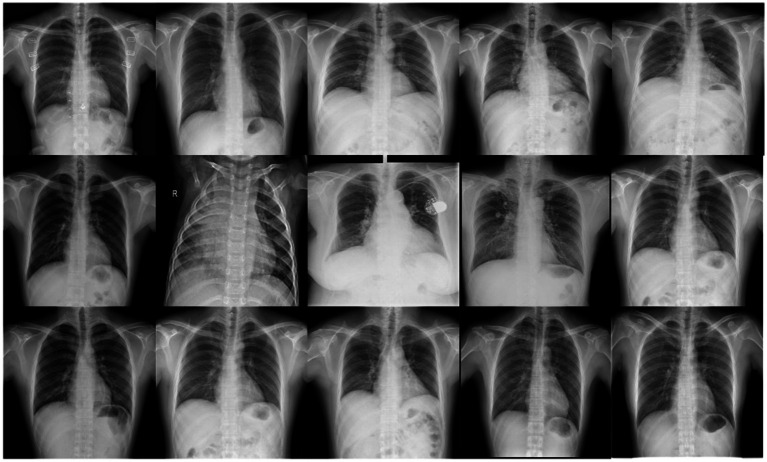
Example images from the NEU-MCD dataset. This figure shows sample chest X-ray images from the NEU-MCD pneumonia detection dataset.
